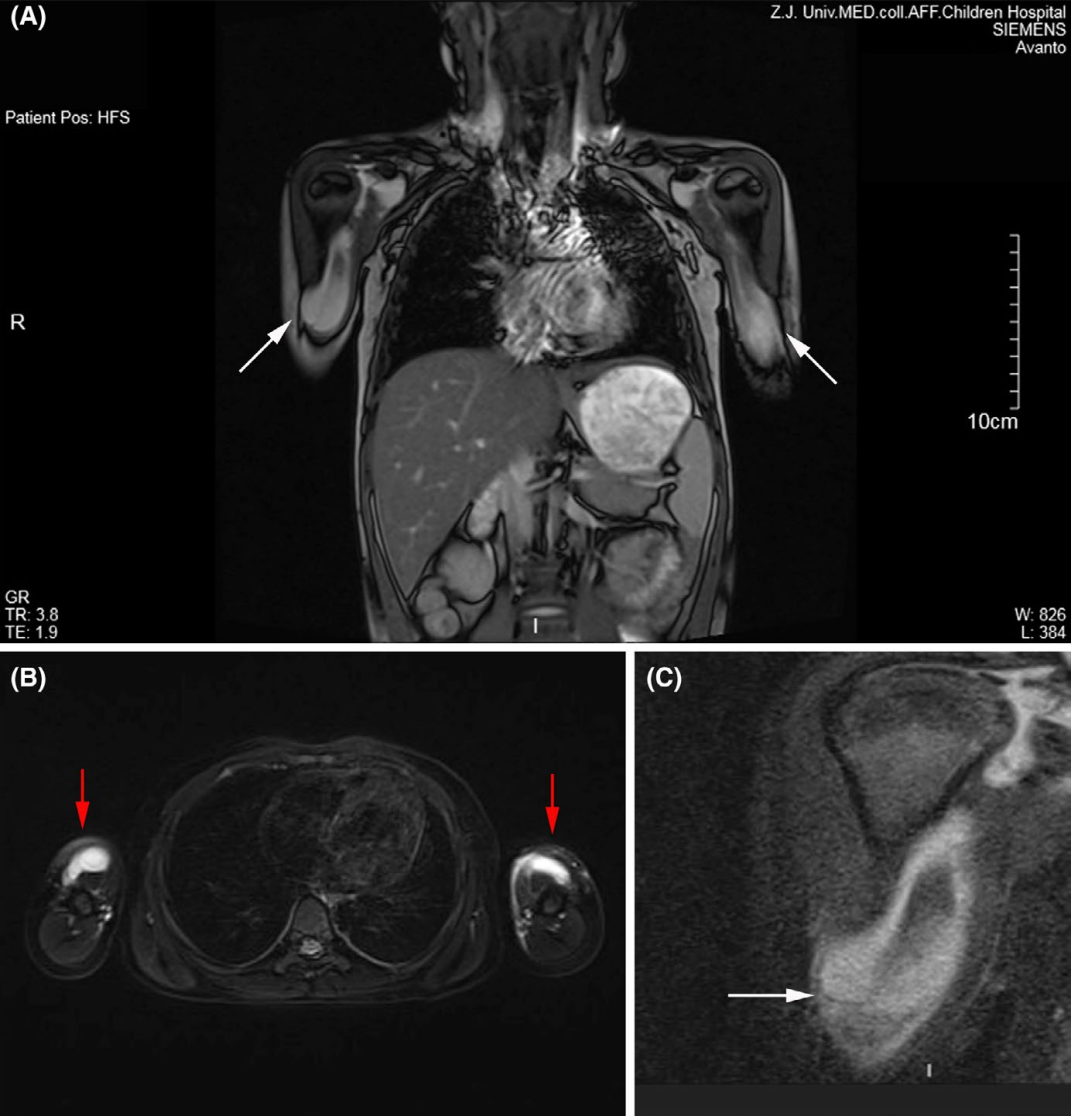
Magnetic resonance imaging (MRI) of bilateral brachial synovial cysts. (A‐C) Longitudinal section of MRI shows irregular cystic fluid signals in bilateral synovial cysts (white arrows). (B) Transverse section of bilateral synovial cysts showing hyperintensity signal on T2‐weighted image (red arrows)
